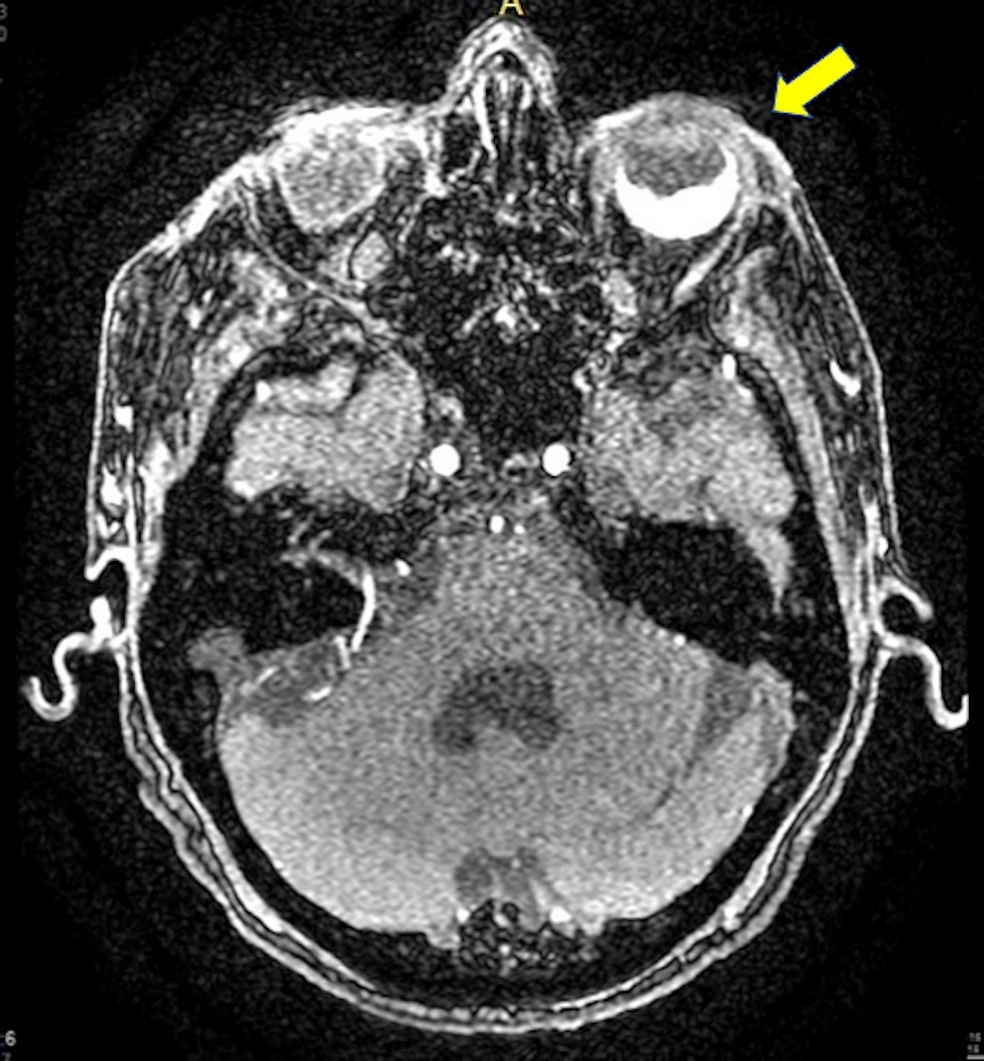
Computed Tomography (CT) of the head (without contrast) demonstrating retinal detachment with evidence of high attenuation material within the left ocular globe
